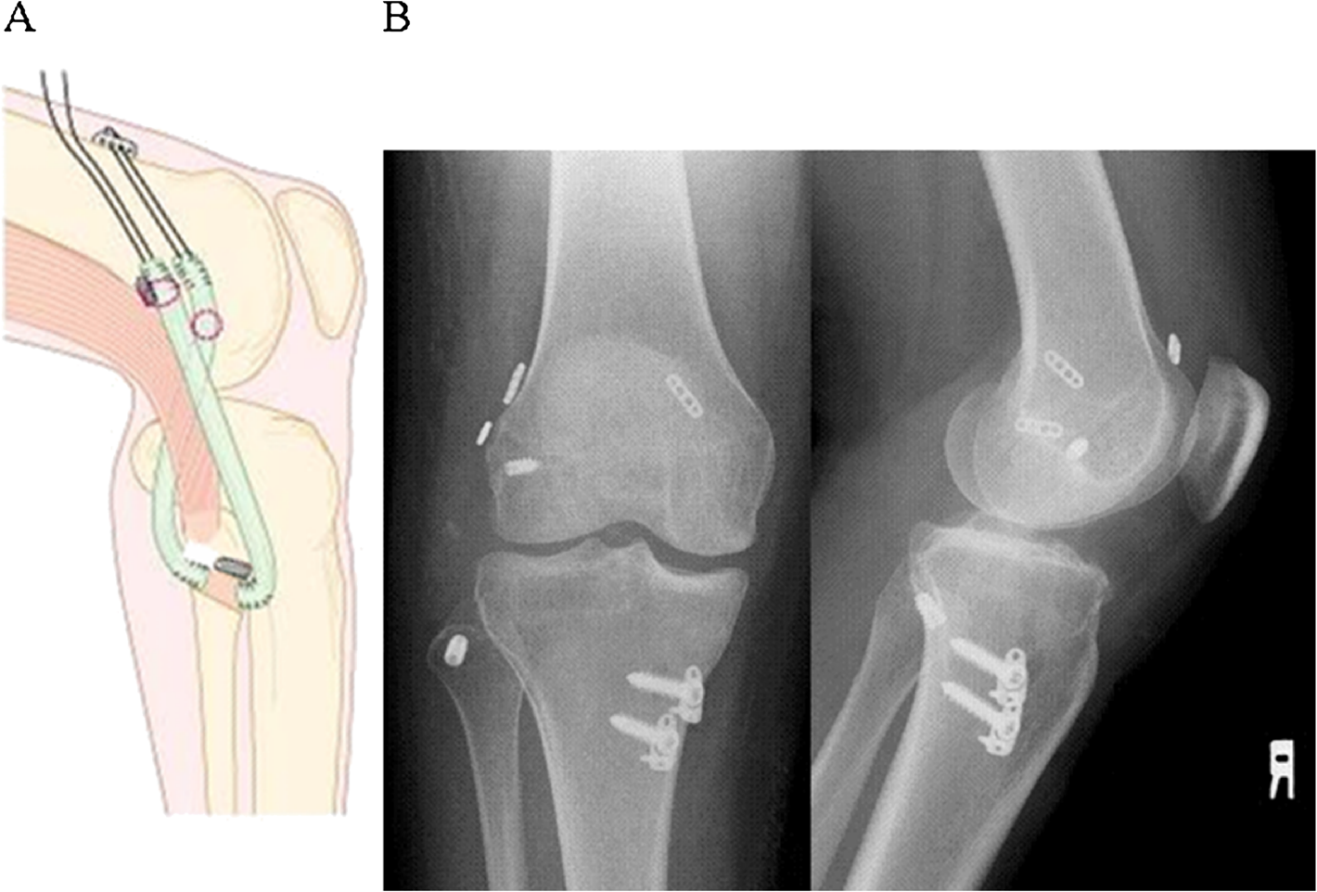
The reconstructed PFL and LCL cross over each other (A). Postoperative radiography shows hardware to be used for securing ACL, LCL, and PFL (B). When either ACL or PCL is reconstructed simultaneously, particularly with a double-bundle technique, great care should be taken with positioning of each bone tunnel to avoid overlap of these tunnels.
